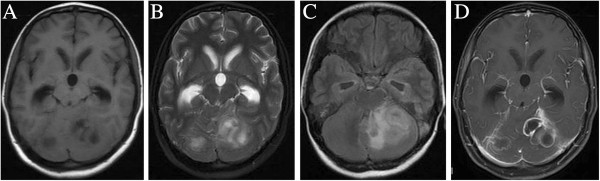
MRI showing Axial T1 hypointense (A), T2 hyperintense (B) signal, DWI (C) and Gadolinium contrast-enhanced (D) image of multiple cerebellar abscesses.
